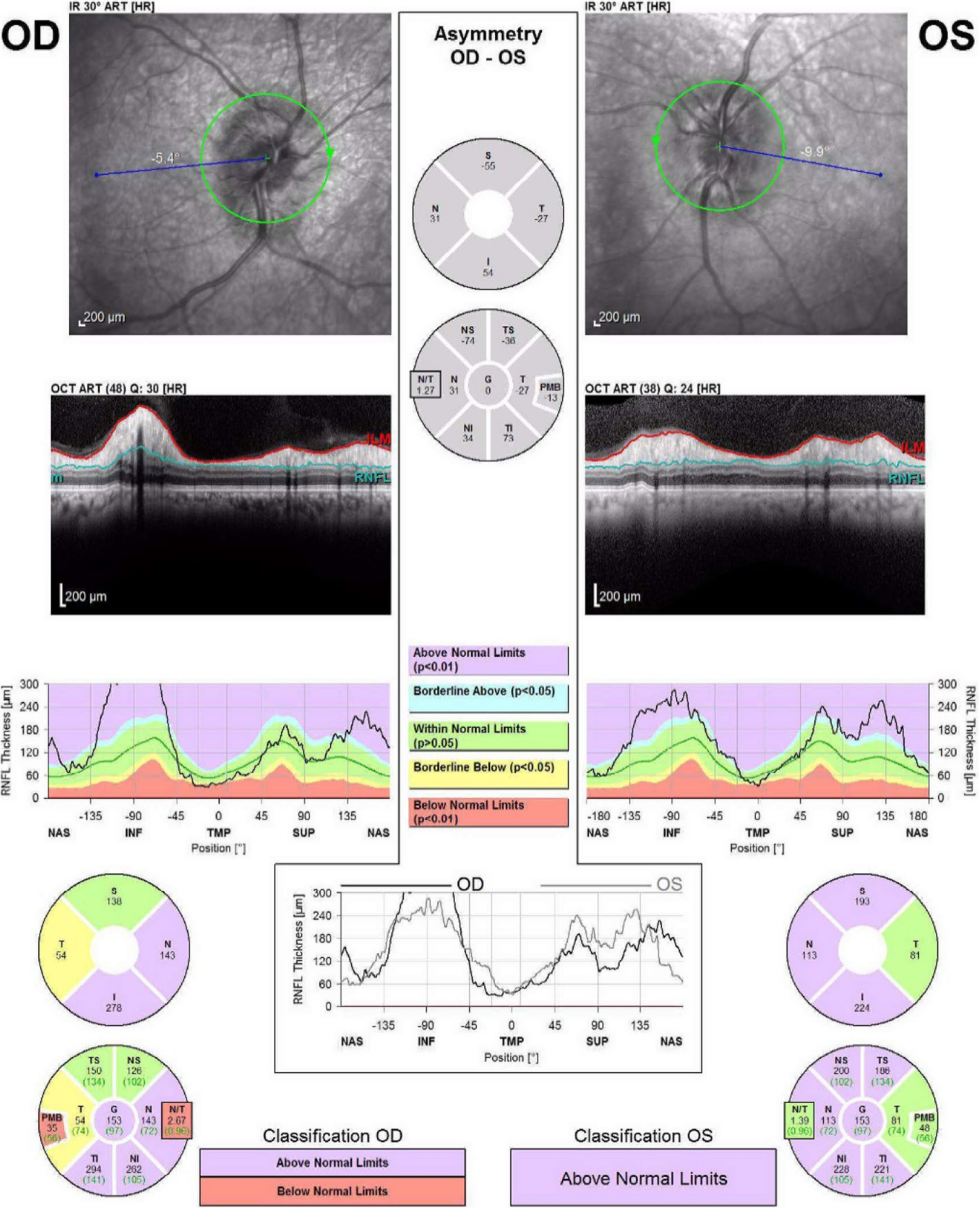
MRI of the orbits shows thickened optic nerve with increased T2 signal and marked enhancement
